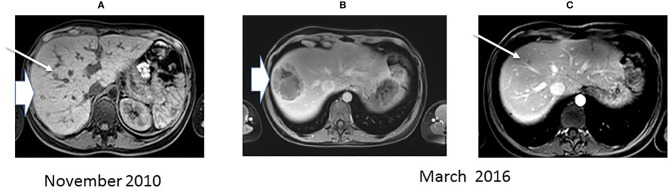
MRI. MRI imaging of the liver in 2010 showing two minute tumors (A one progressed (arrowhead) B) in March 2016 while the other (thin arrow) (C) remained stable.
